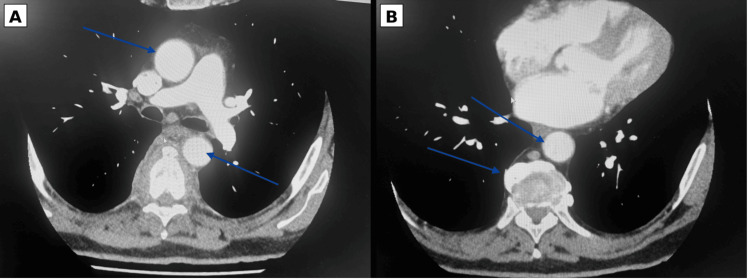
CT Angio CT angiography of the aorta, 23 December 2025. (A) Axial section at the aortic arch and great vessels. The aorta is well opacified with no wall thickening or periaortic fat stranding. (B) Axial section at the level of the affected vertebral segment, with the opacified descending thoracic aorta adjacent to the vertebral body. There is interval disc height loss and pre- and paravertebral soft tissue change in keeping with discitis and osteomyelitis (reported at T7–T8), but no mycotic aneurysm, aortitis, or epidural abscess. The affected vertebral level was reported as T7–T8 on CT angiography and T6–T7 on MRI; this difference reflects the lower spatial resolution of CT for early endplate change rather than progression of disease
